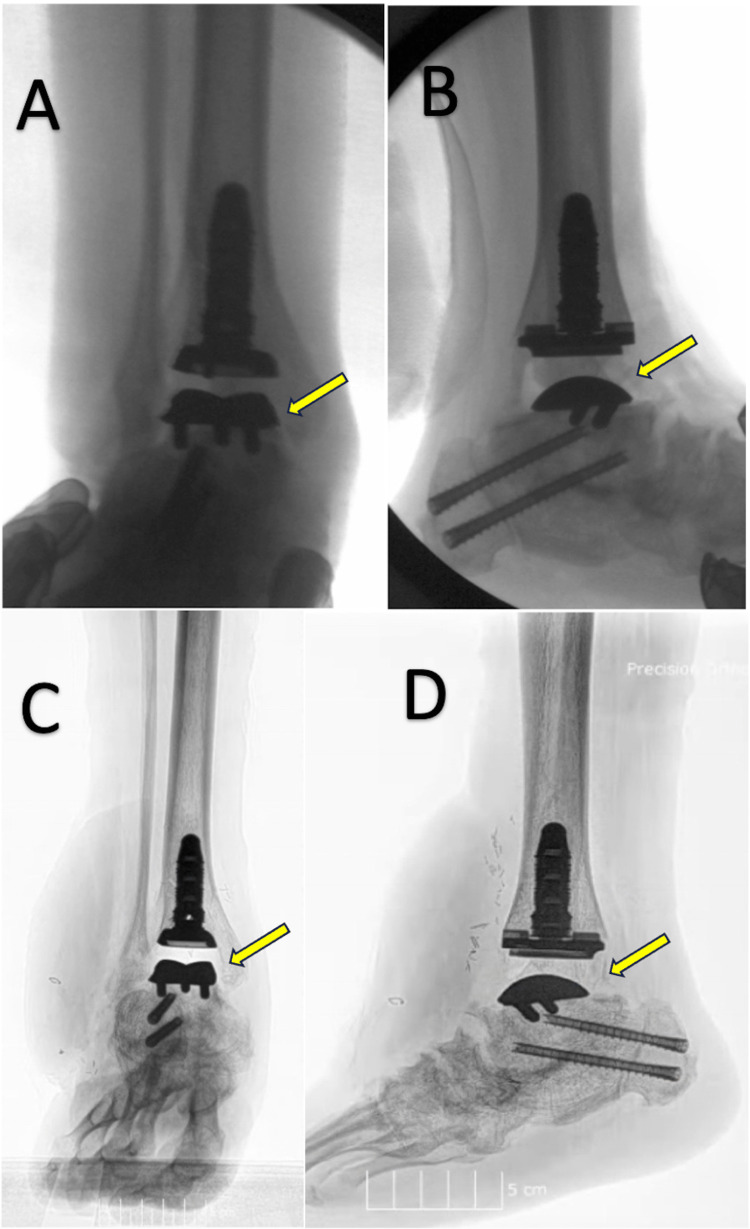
Radiographs of the patient’s right ankle after TAA and free flap microsurgery. (A) Posterior-anterior X-ray view. (B) Mediolateral X-ray view. (C) Anterior-posterior X-ray view. (D) Lateromedial X-ray view. TAA, total ankle arthroplasty
